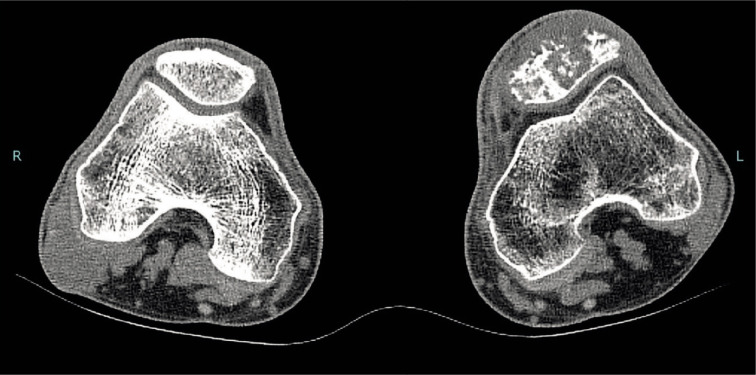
A CT scan that shows a degeneration of the left patella.
